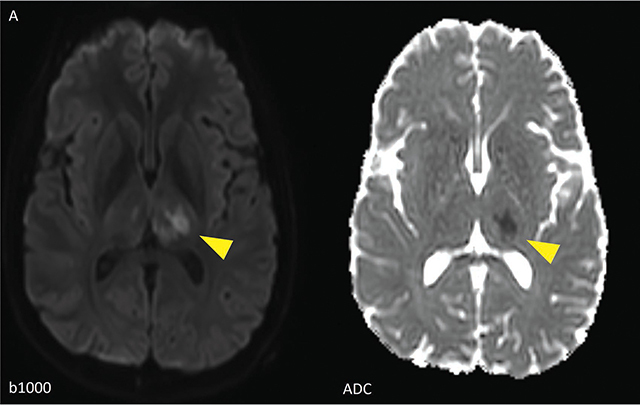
DWI images showing multiple areas of diffusion restriction, corresponding with acute ischemia (yellow arrowheads) in the left thalamus.
